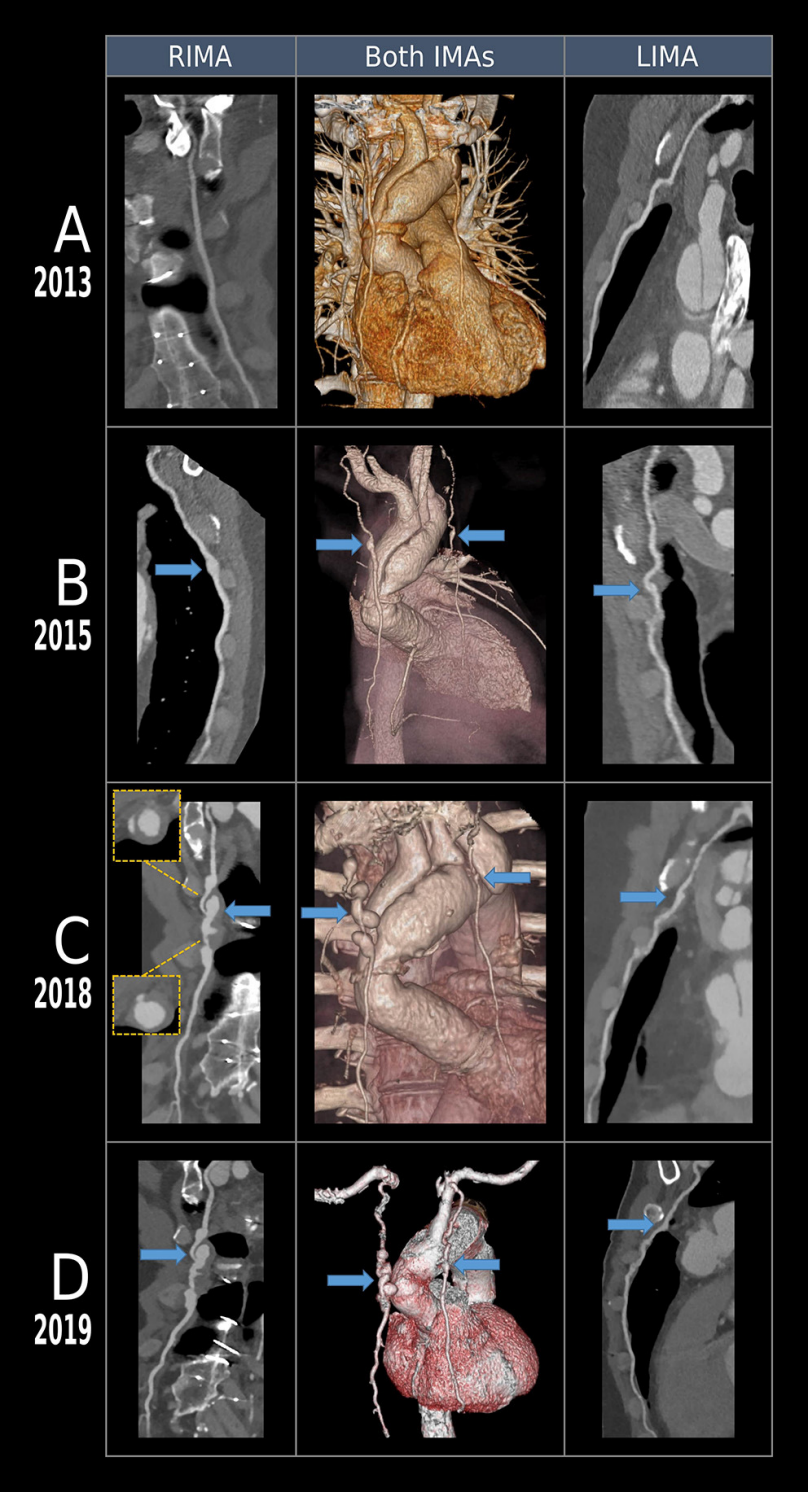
Volumetric and multiplanar reconstructions of the aorta, left and right internal mammary artery (IMA) of a Marfan syndrome patient after Bentall procedure. 256-slice CT angiography of the chest was performed at three different timepoints. (A) In 2013, left and right IMA-s were intact without any signs of aneurysms. (B) In 2015, both arteries demonstrated a small dilatation (~0.5 cm) in the mid-section. (C) Follow-up scans after 3 years revealed a saccular pearl-string-like aneurysm of the right IMA with thrombus on the walls, an aneurysm of the left IMA and increase in diameter of the aortic arch. (D) Follow-up CT done in 2019 shows no progression of the aneurysms.
